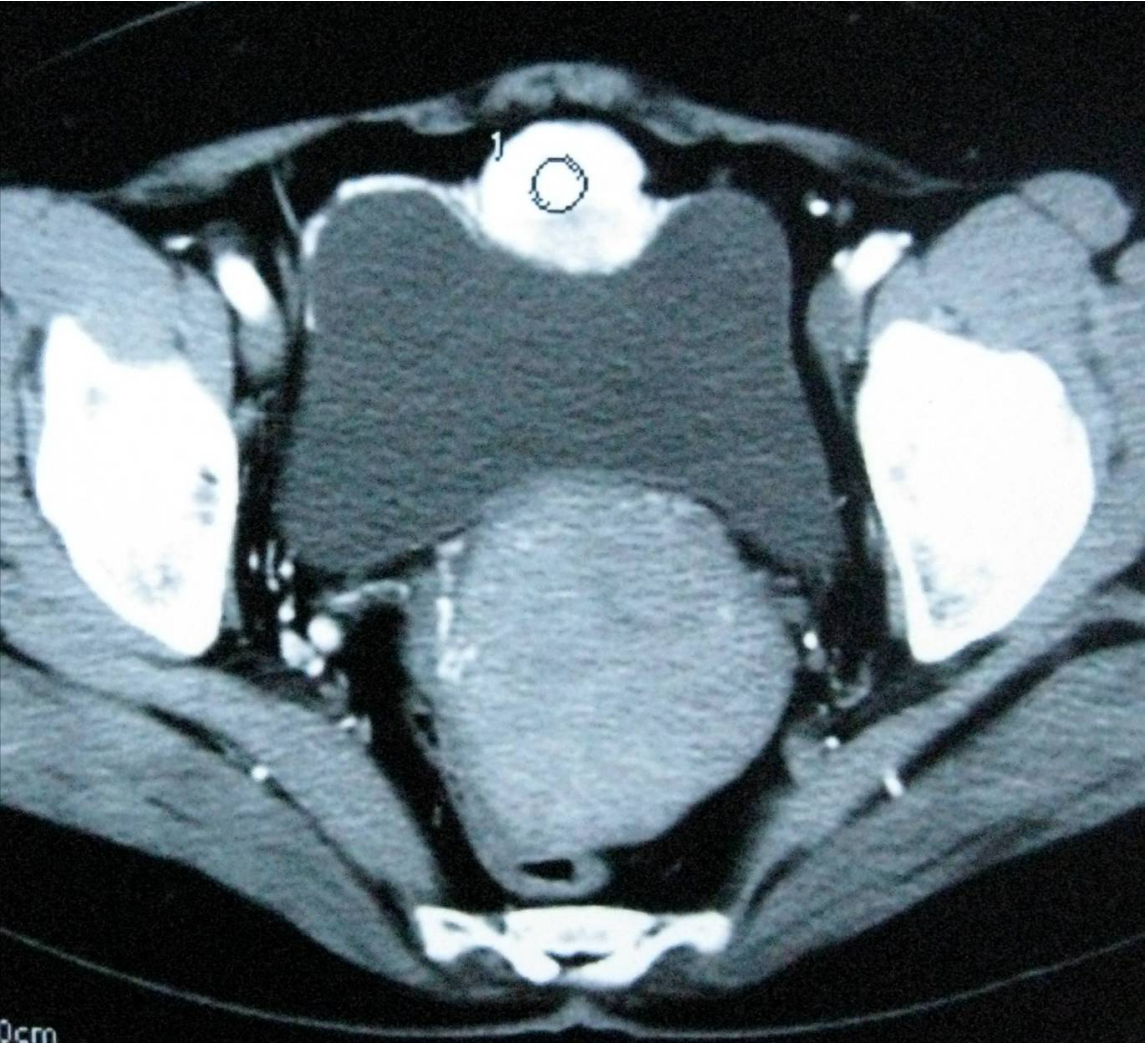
CT scan showing a nodular mass with intensive enhancement on the dome of bladder.
